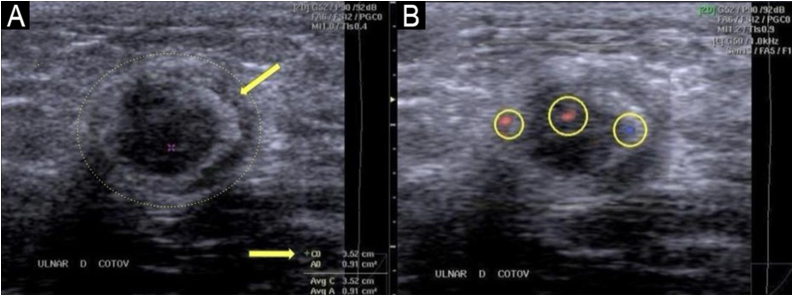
Ultrasonography of the ulnar nerve (transverse plane at the elbow). (A) B-mode imaging reveals marked thickening with a perimeter of 3.52 cm and an area of approximately 0.91 cm^2^ (≈91 mm^2^); for reference, normal is approximately 0.10 cm^2^ (≈10 mm^2^). Note epineurial thickening and a crescent-shaped hyperechoic halo (arrow). (B) Color Doppler at the same level shows three foci of peri-/intraneural flow (circles 1–3) within the Doppler box, consistent with inflammatory neuritis.
